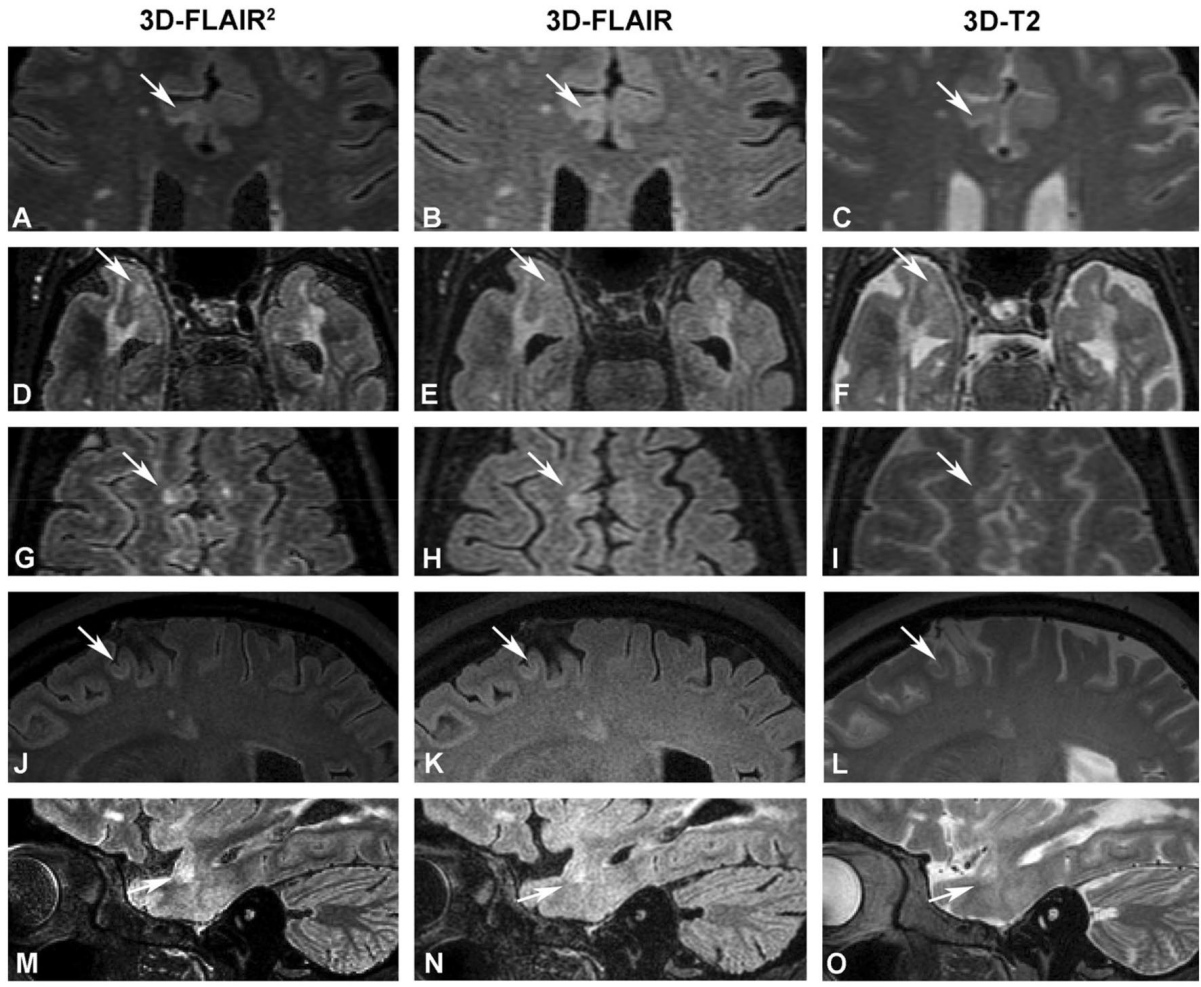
3D-FLAIR^2^ lesion visualization compared to 3D-T2 and 3D-FLAIR. A-C: Depiction of a cortical/leucocortical lesion on axial 3D-FlAIR^2^ (A), 3D-FLAIR (B), 3D-T2 (C) MRI images. D–F Presentation of a large white matter lesion with an adjacent cortical/leucocortical lesion. G–I Juxtacortical lesion involving the right frontal superior gyrus; J–L Juxtacortical U-fiber lesion on sagittal view. M–O Temporal cortical/leucocortical lesion on sagittal view
